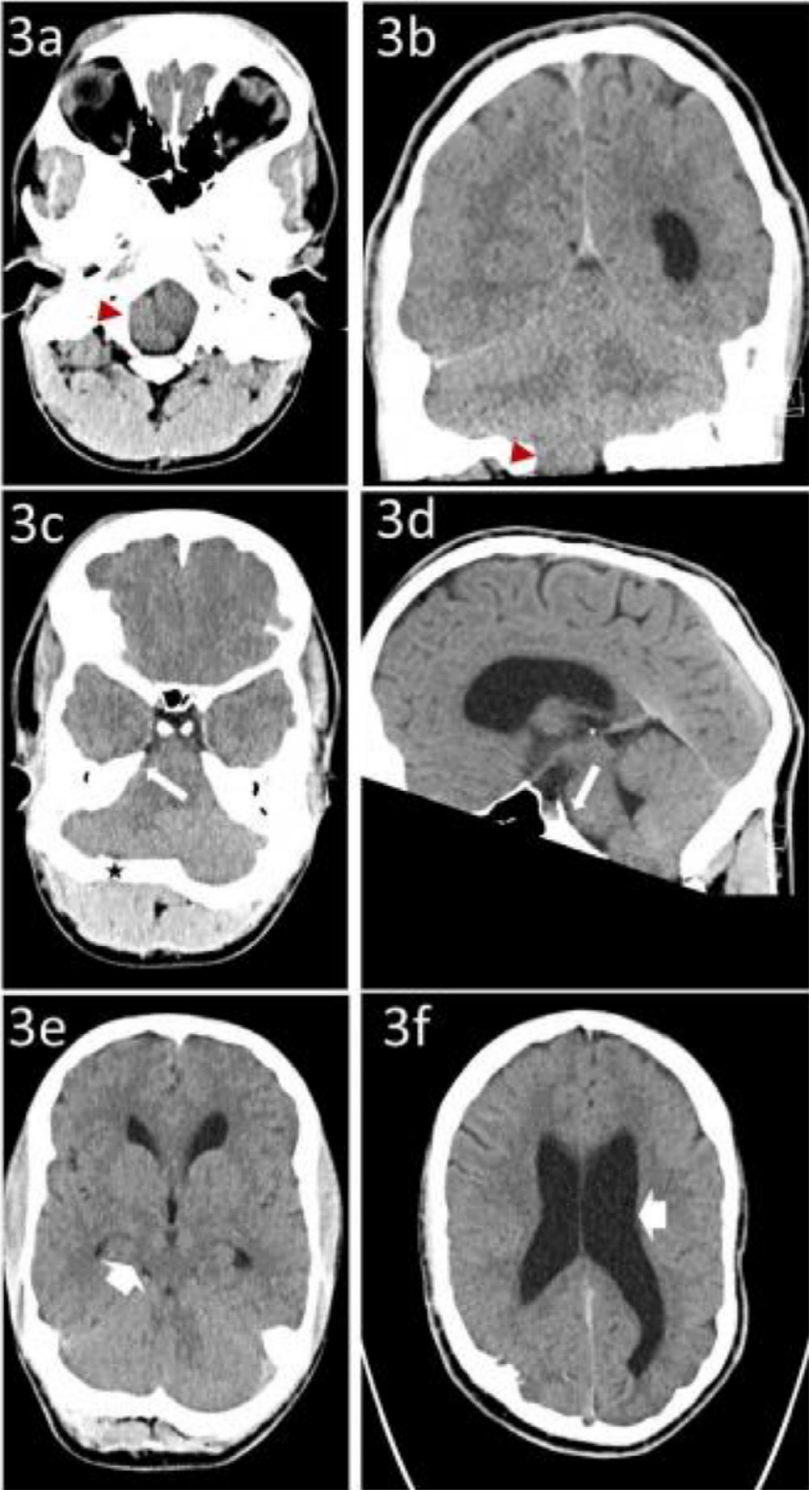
A 21-year-old male with a known Pfeiffer Syndrome presented following trauma. Brain CT without IV contrast (3A), (3C), and (3E) in the axial plane. (3B) in the coronal plane and (3D) in the sagittal plane. (3A) and (3B) demonstrate crowding of the foramen magnum with extension of the right cerebellar tonsil through the foramen magnum, red arrow head. (3C), and (3D) shows prepontine cistern effacement, white long arrow. (3C) shows narrowing of the occipital fossa, black star. (3E) quadrigeminal cistern effacement is demonstrated marked by the short white arrow. (3F) shows bilateral enlargement of the lateral ventricles, short white arrow.
